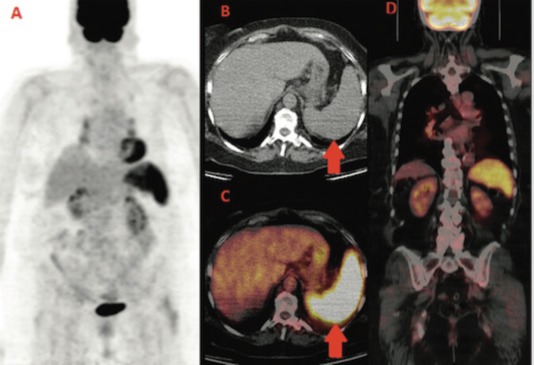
First F-FDG performed; intense and diffuse tracer uptake in the spleen and hilar lymph nodes.
